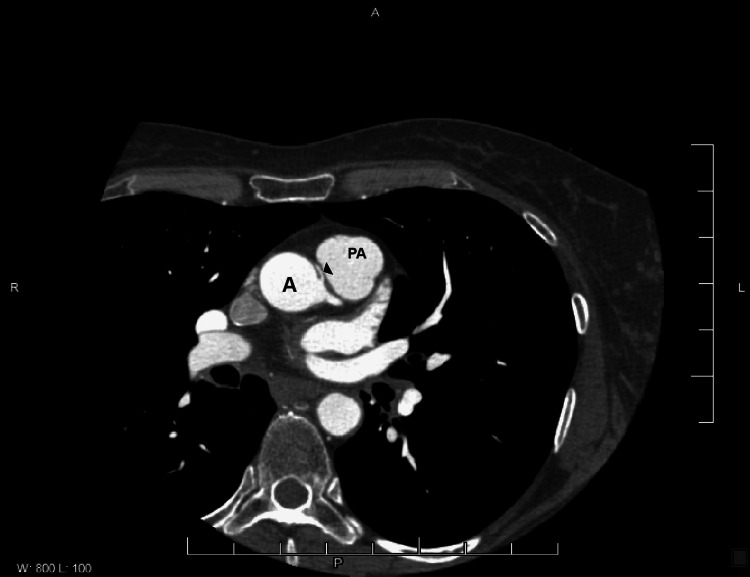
CT cardiac angiography showing anomalous origin of right coronary artery from left cusp (arrowhead 🢐). The RCA then exhibits an inter-arterial course between the aorta (A) and the pulmonary artery (PA).
